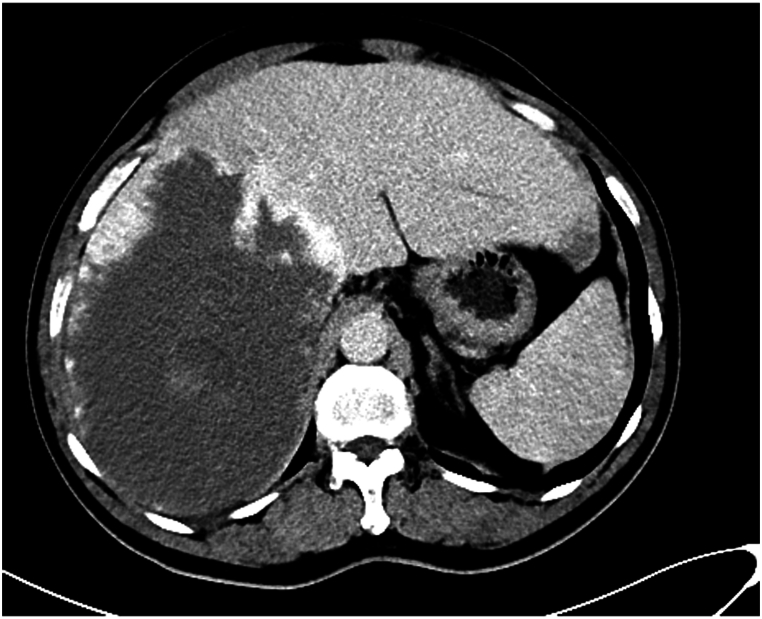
CT image obtained before DEB-TACE revealed a 187.5 × 142.7 mm CHL in the right lobe of the liver.
